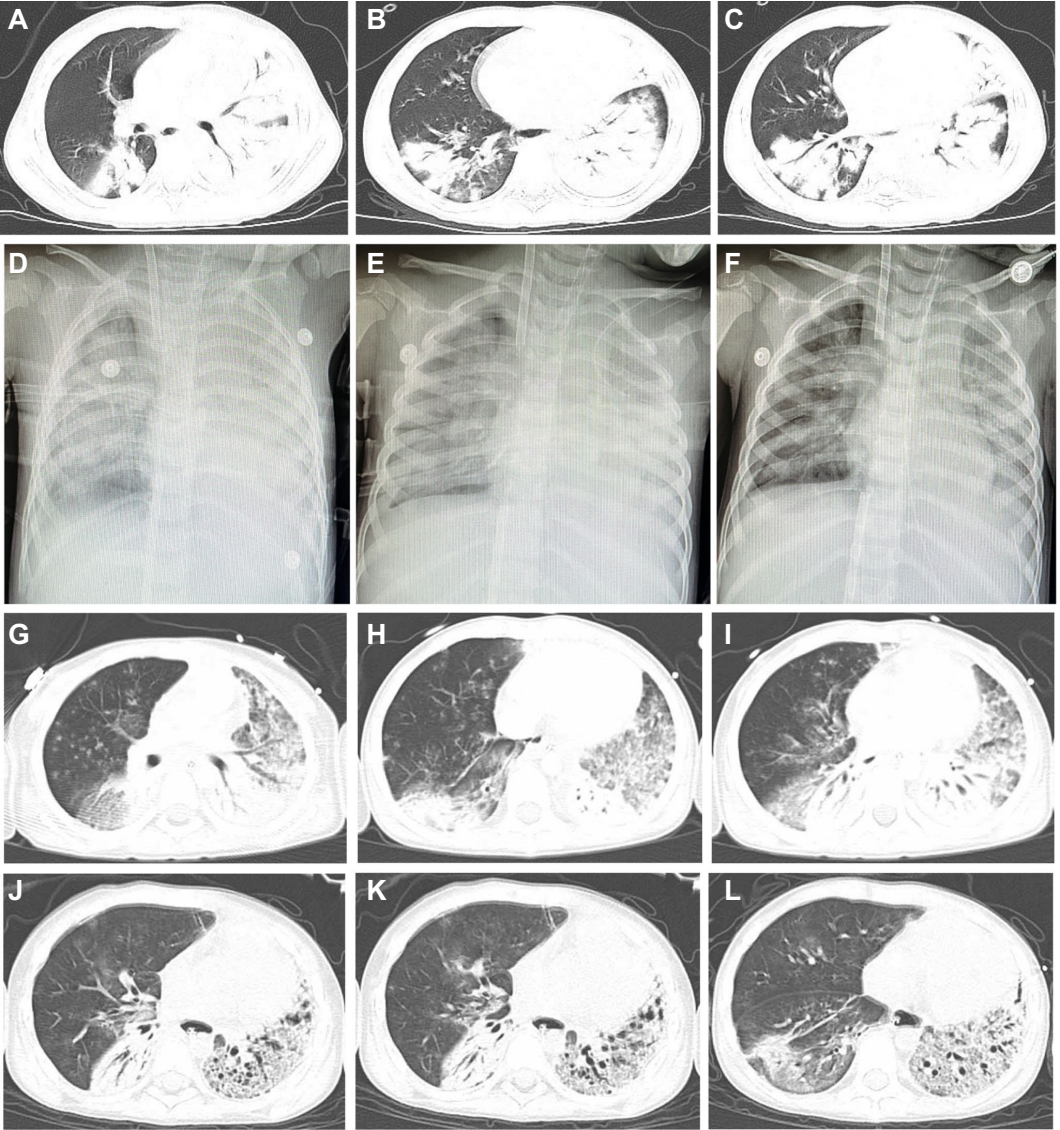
Imaging of the patient’s chest. Computed tomographic (CT) scan obtained on day 6 of illness (A–C). CT scan showed multiple patchy high-density shadows in both lungs, especially in the lower lobe of the right lung and the left lung. Chest radiographs showed patchy high-density shadows in both lungs on day 7 of illness (D). After treatment 2 days, the pulmonary shadows were absorbed slightly on day 8 of illness (E) and on day 9 of illness (F). The consolidation shadow in the left lung was more absorbed than before on day 16 of illness (G–I) and day 31 of illness (J–L).
